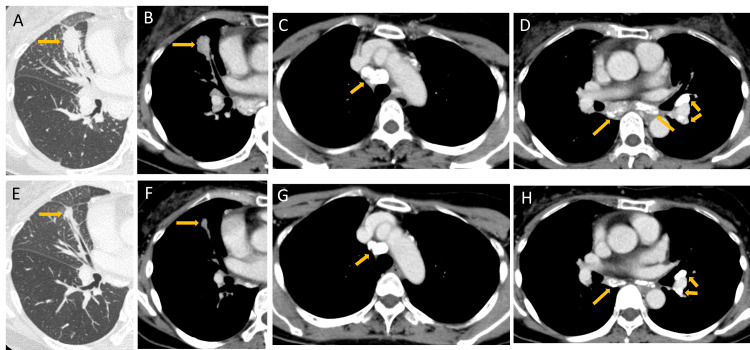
CT (A, B) CT showing a 25 × 14 mm nodular shadow in the right S5 lobe. (C, D) Hilar and mediastinal lymph nodes exhibiting extensive calcification. (E-H) Changes observed after one month of ROS1/MET inhibitor therapy. CT, computed tomography
